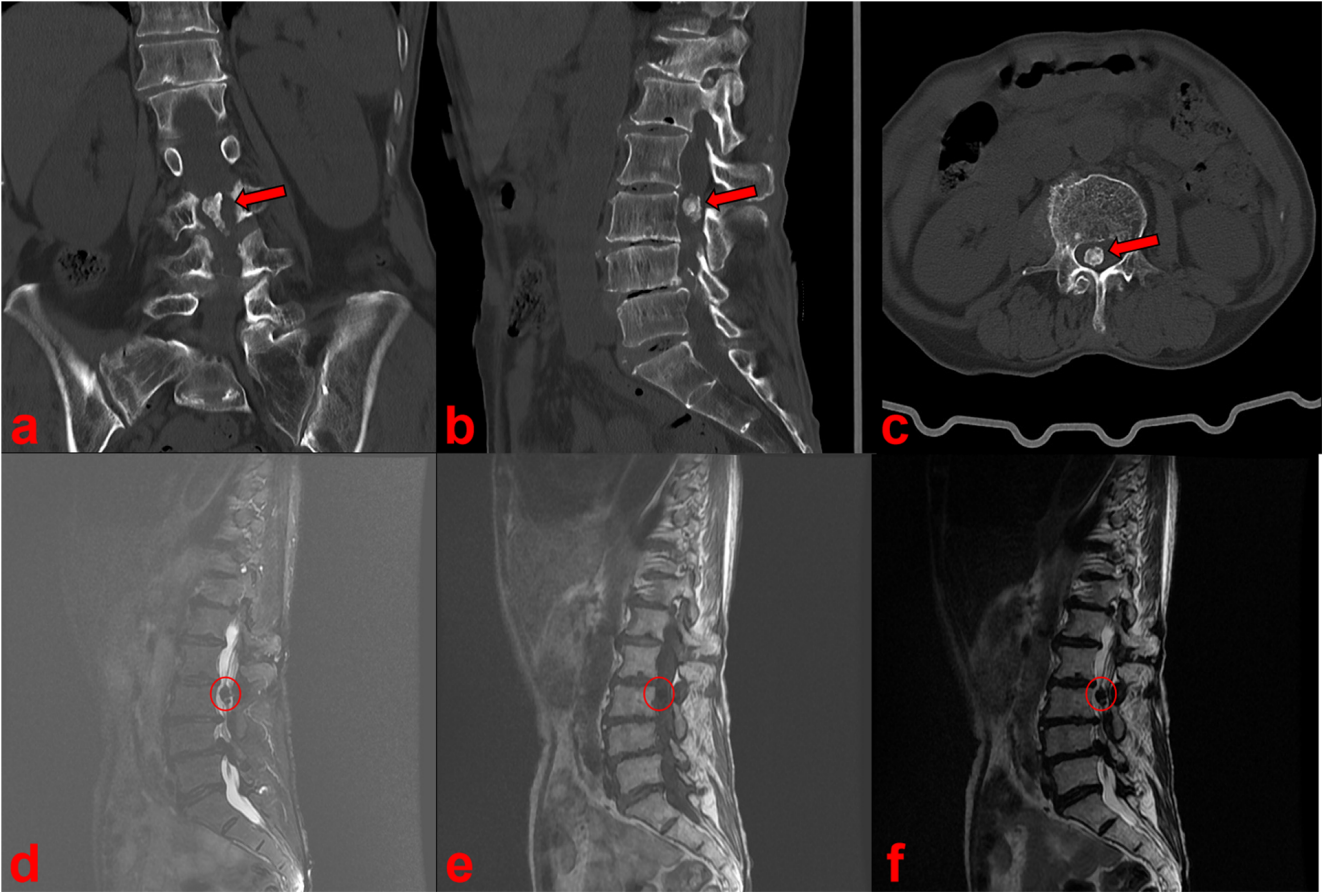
CT (a–c) and MRI (d–f) scan showed a calcified intradural lesion at the L3 vertebral level.
